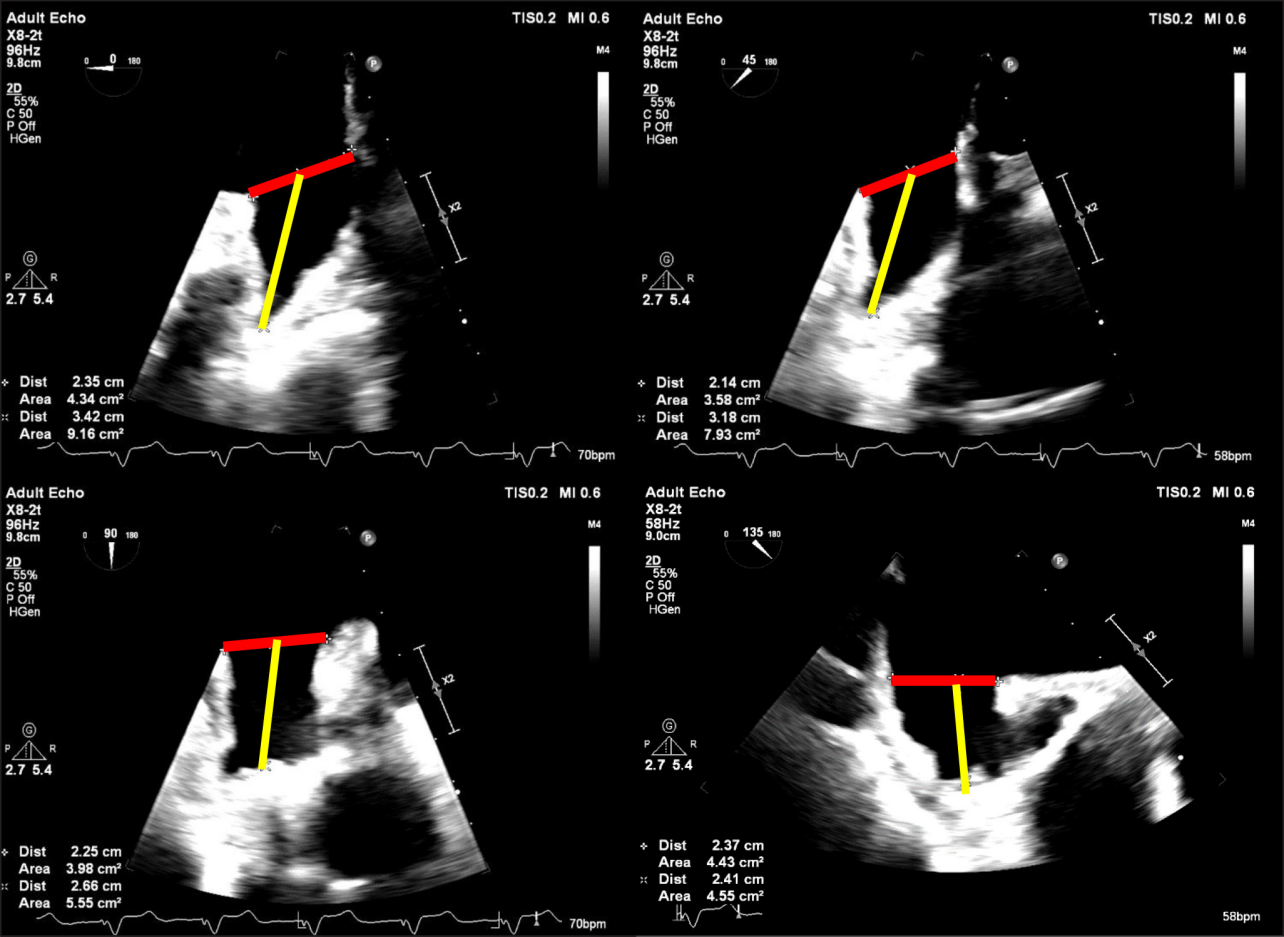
Transesophageal echocardiogram demonstrating left atrial appendage measurements on different planes (0, 45, 90 and 135 degrees). The horizontal red line represents the width and the yellow line represents depth.
